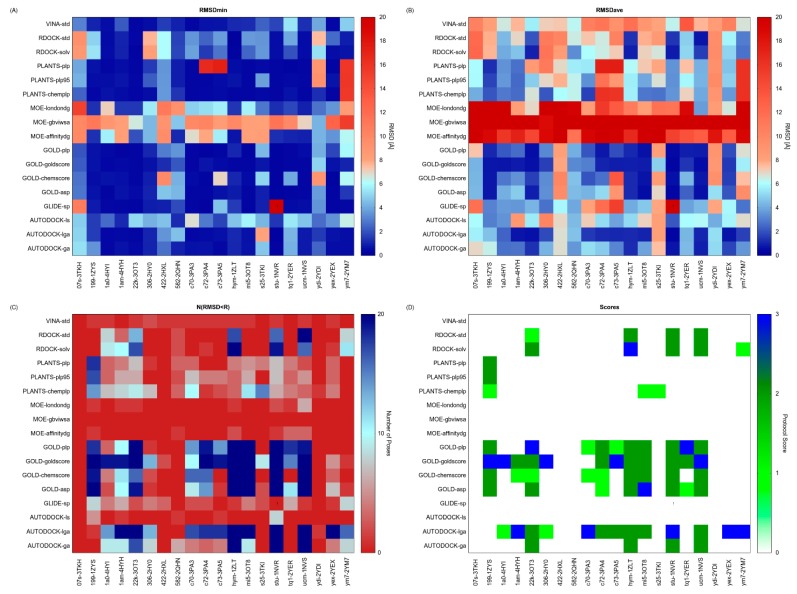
Results of the docking benchmark study on human checkpoint kinase 1. (A) Minimum RMSD values (RMSD_min_) returned by the tested docking protocol (y-values) for the considered X-ray structures (x-values); (B) Average RMSD values (RMSD_ave_); (C) Numbers of conformations returned by each docking protocol having a RMSD value lower than the X-ray structure resolution (N^(RSMD < R)^); (D) Protocol Score. RMSD is expressed in Å, whereas the Protocol Score on a 0–3 points scale. Values are rendered with a colour code, blue spots identify the best obtained results. Exclamation marks warn that an error occurred during docking calculations.
